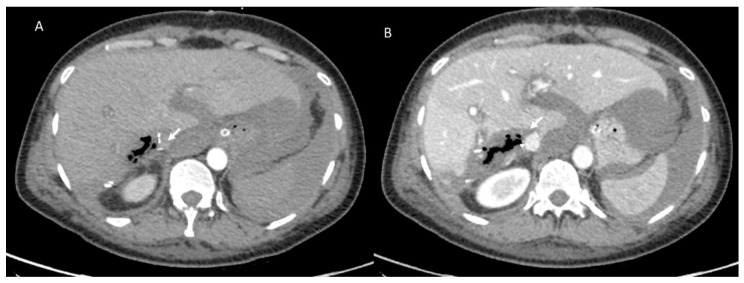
Hepatic abscess in resected hepatocellular carcinoma on VI hepatic segment, evaluated with CT. Arrow shows air artifacts within the collection in arterial (A) and portal (B) phase of contrast study.
